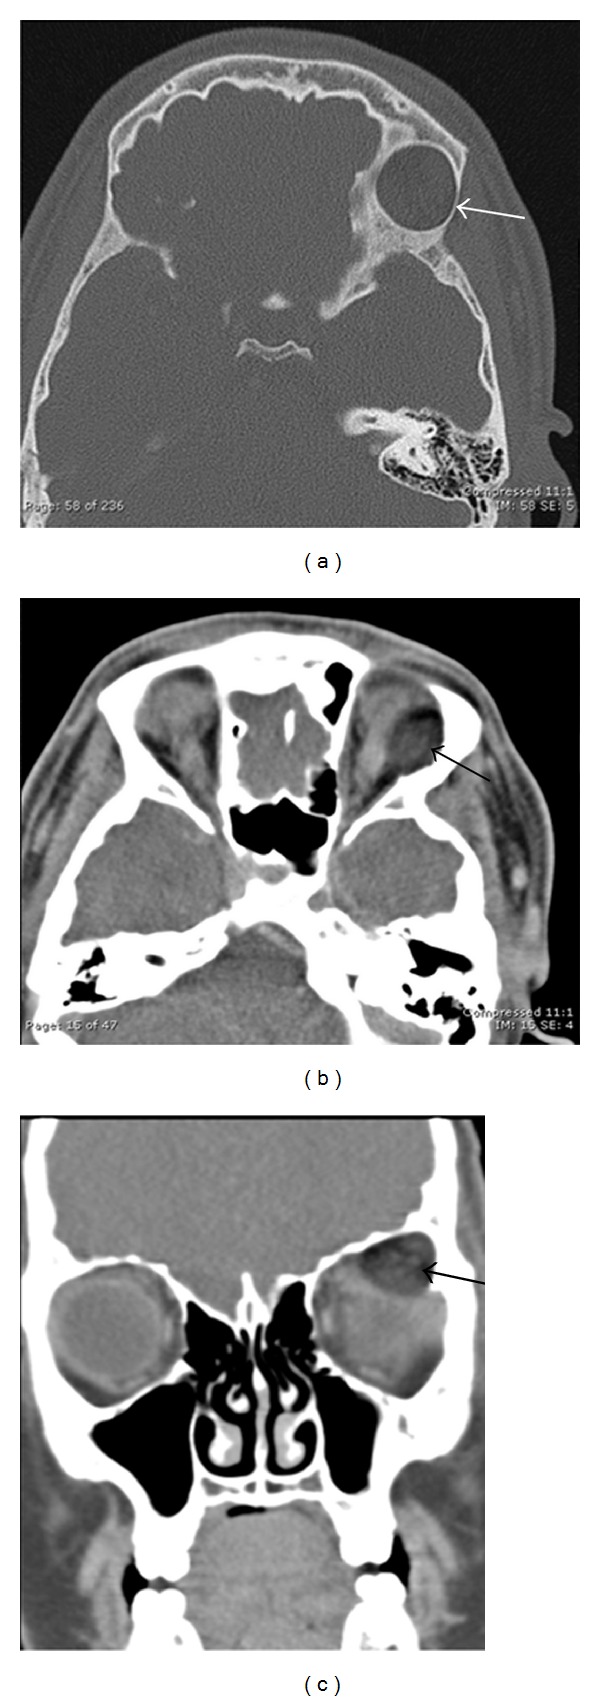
18 years old with diplopia. Axial CT with bone algorithm (a) shows a well-circumscribed lesion in the superolateral left orbit that causes adjacent bone thinning (white arrow) and sclerosis. Axial (b) and coronal (c) CT with soft-tissue algorithm show low attenuation fat within the mass (black arrows), diagnostic of a dermoid inclusion cyst.
